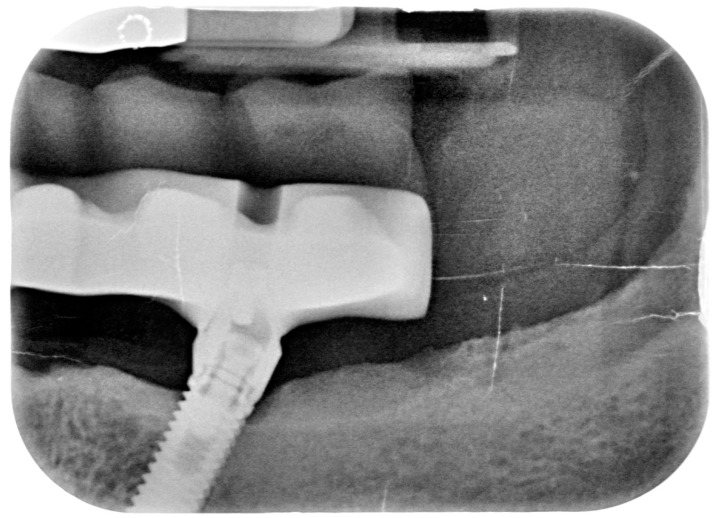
X-ray of 3.5 dental implant in patient before treatment.
